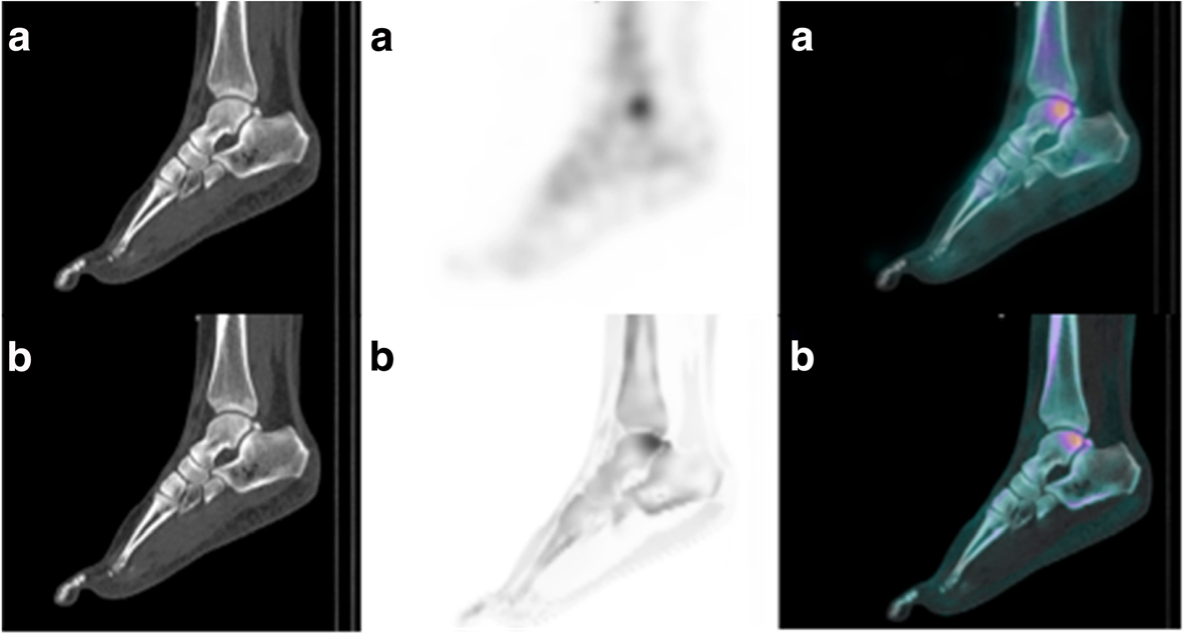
Example of a better location of a pathological uptake with xSPECT Bone® reconstruction. This SPECT/CT was performed in order to explore a chronic right ankle pain which appeared 1 year ago without traumatism. An uptake of the talus is observed on the F3D image (a) whereas an uptake of the talus and of a trigonum bone is observed on the xB image (b). MRI realized after SPECT/CT did not show contusion of the talus but confirmed the right os trigonum syndrome observed on xB image
